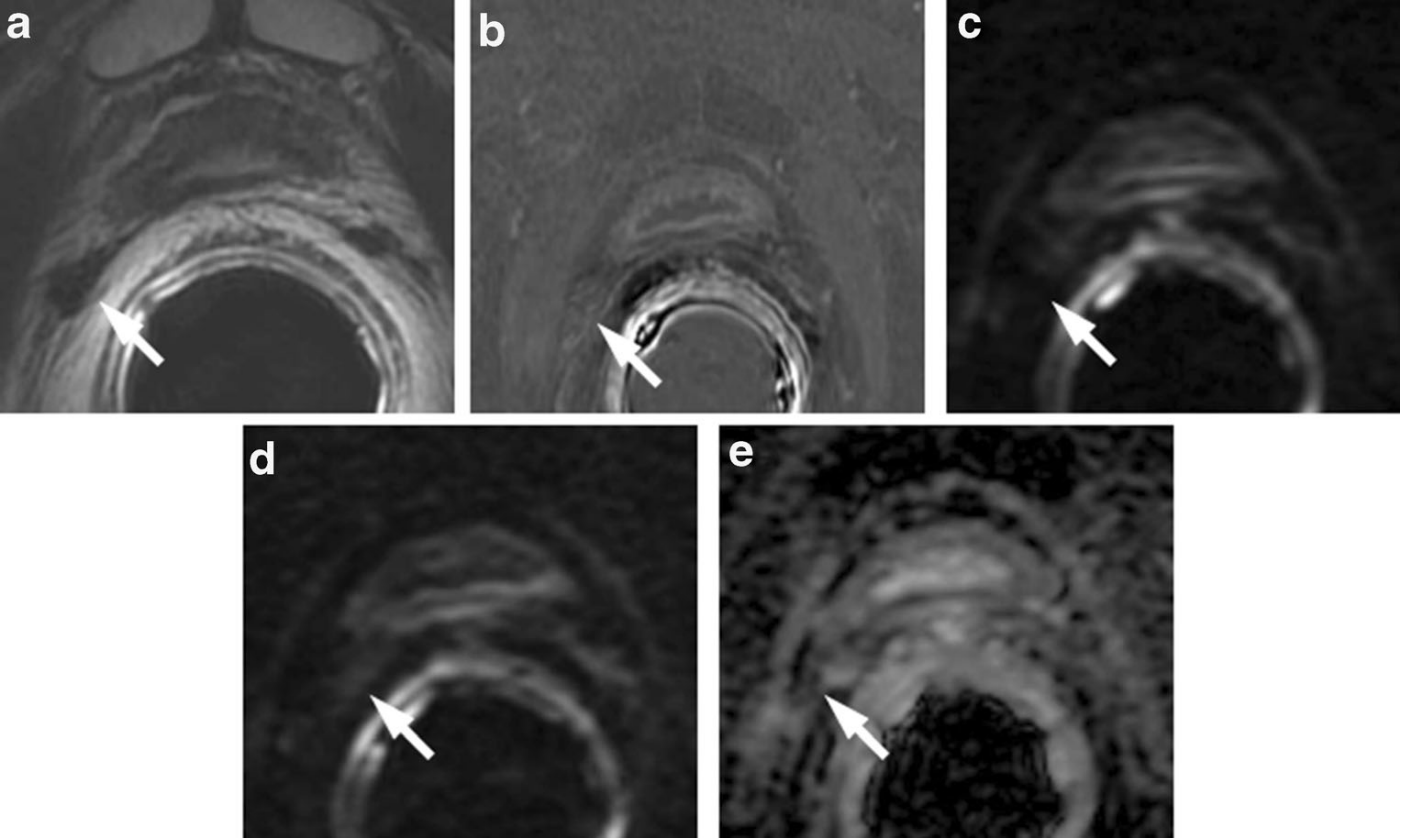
MR images of a 64-year-old man with prostate-specific antigen progression (PSA serum level 0.9 ng/mL) after radical retropubic prostatectomy, with suspected local recurrence. a Axial T2-weighted fast spin-echo image shows, on the zone of right seminal vesicle, a nodular tissue (*white arrows*) with signal intensity similar to that of muscle. b Axial gradient-echo T1-weighted subtracted image shows no signs of enhancement on the same location as the solid nodular tissue seen on trasverse T2-weighted images. c Diffusion weighted images with a b value of 1.000 and d 3.000 s/mm^2^ and e ADC map show no diffusion restricted phenomena on corresponding site. These findings are consistent with seminal vesicle remnants with fibrotic evolution
